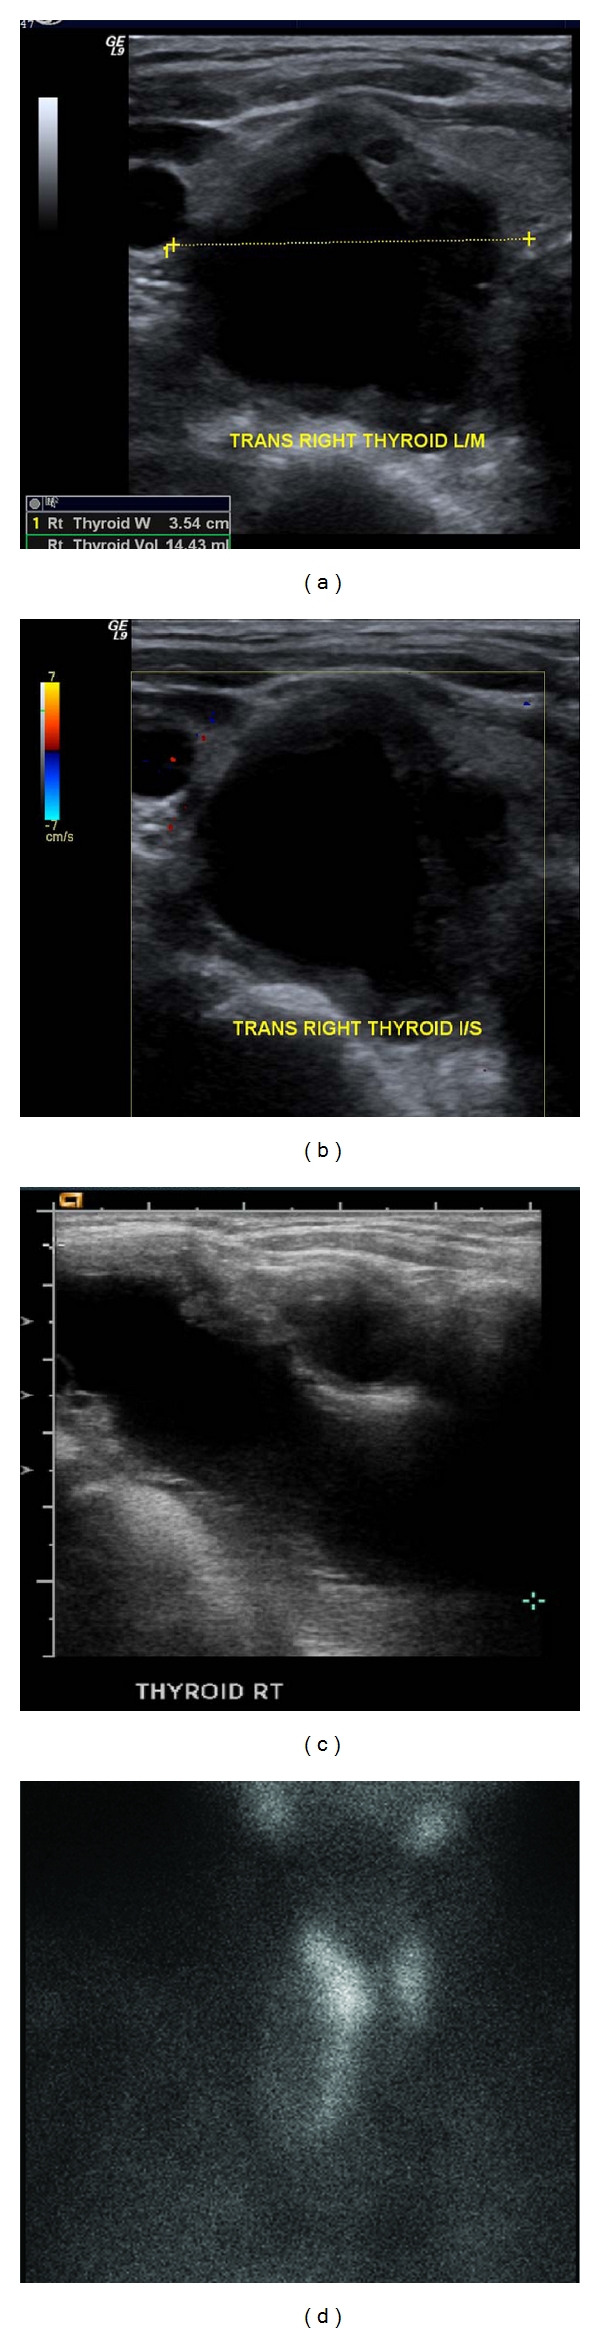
(a, b, c) Thyroid ultrasound showing large complex cystic mass within or adjacent to the right lobe of the thyroid gland measuring up to 4.5 cm representing thyroid versus parathyroid lesion. (d) Parathyroid scan shows increased uptake in the lobes of thyroid gland. “MIBI avid lesion with retained activity in the periphery.”
